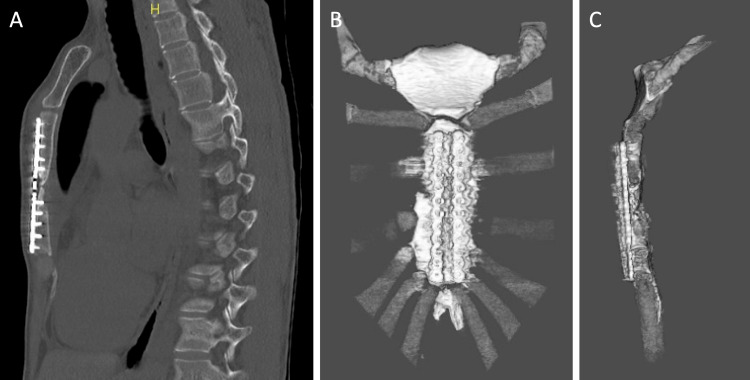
Postoperative images of sternal fracture (A) Sagittal CT image; (B) Anterior view of three-dimensional reconstructed CT image; (C) Lateral view of three-dimensional reconstructed CT image CT, Computed tomography
